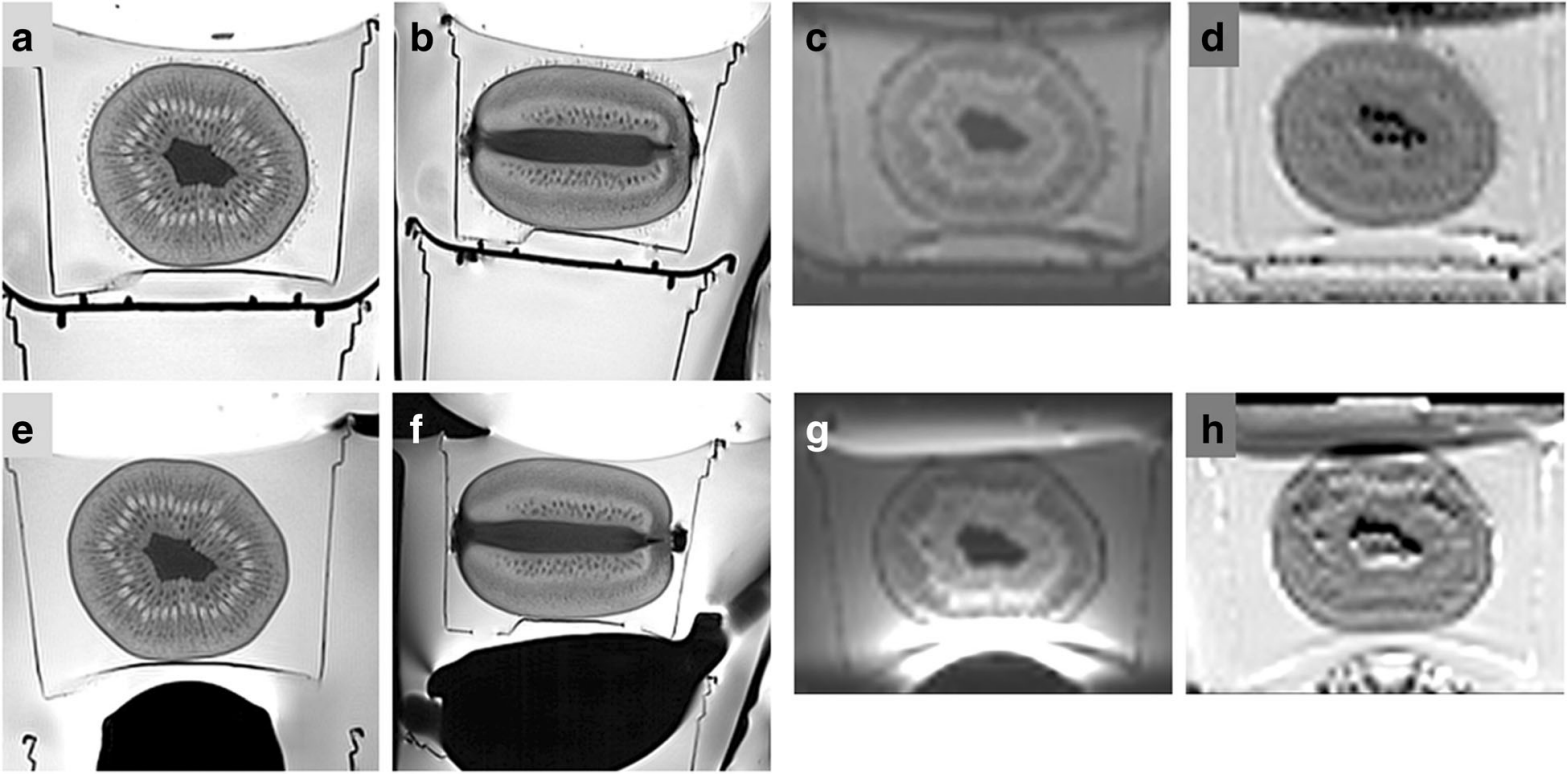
Biparametric magnetic resonance imaging at 3.0 T of a green Hayward kiwifruit (*Actinidia deliciosa*) was performed at site 1 both without (a–d) and with an endo-rectal coil (e–h) included in a multichannel phased-array coil system: axial (a, e) and sagittal (b, f) T2-weighted imaging (T2WI), axial diffusion-weighted imaging with a *b* value of 800 s/mm^2^ (c, g), and corresponding apparent diffusion coefficient map (d, h) obtained with a reduced field of view
